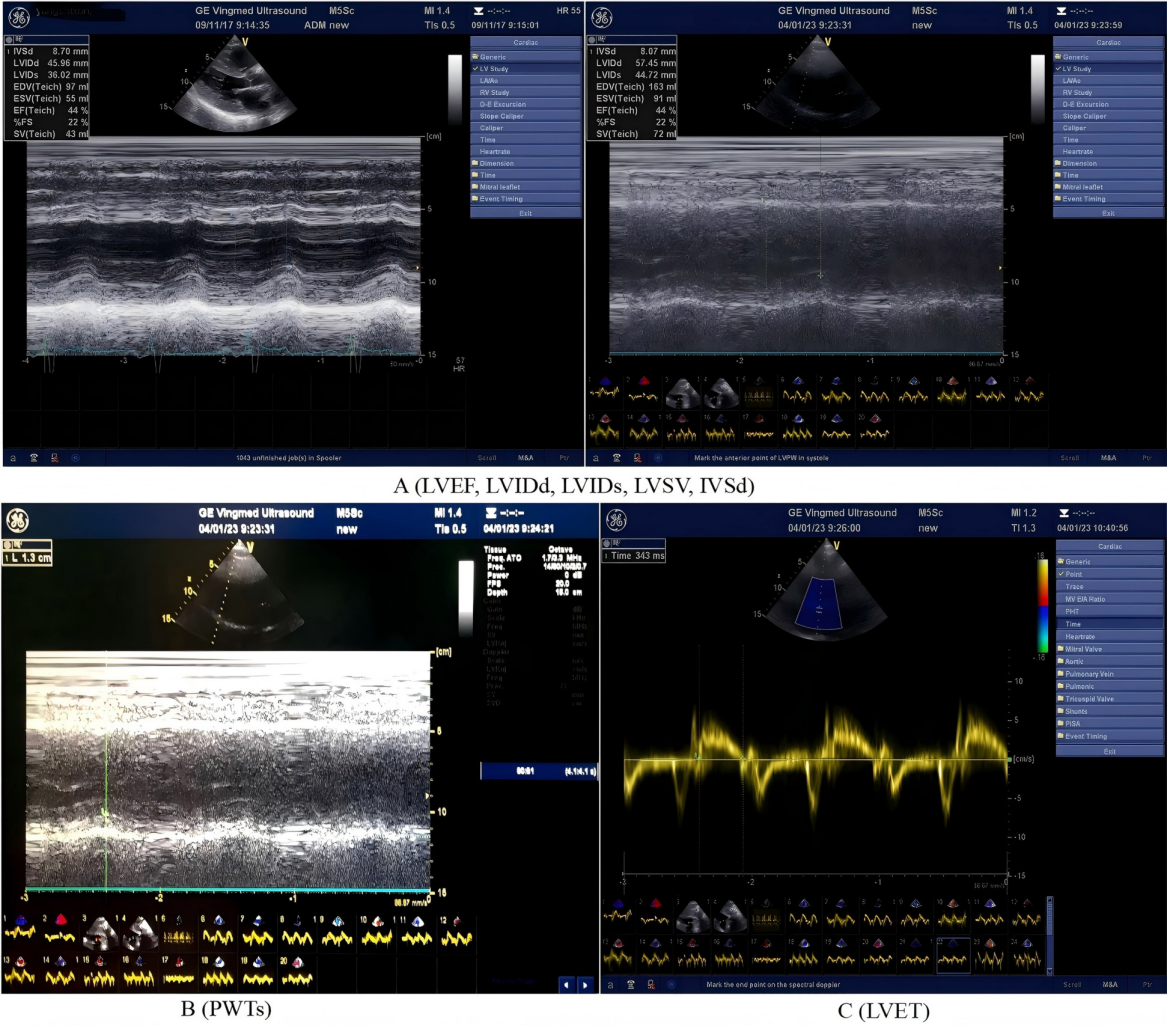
Echocardiographic parameters (including left ventricular structural, functional, volumetric and ejection time) and measurement methods. The panel A (LVEF, LVIDd, LVIDs, LVSV, and IVSd): LVEF, left ventricular ejection fraction; LVIDd, left ventricular internal diameter in diastole; LVIDs, left ventricular internal dimension in systole; LVSV, left ventricular stroke volume; IVSd, interventricular septal thickness in diastole. The panel B (PWTs): left ventricular posterior wall systolic thickness. The panel C (LVET): left ventricular ejection time.
